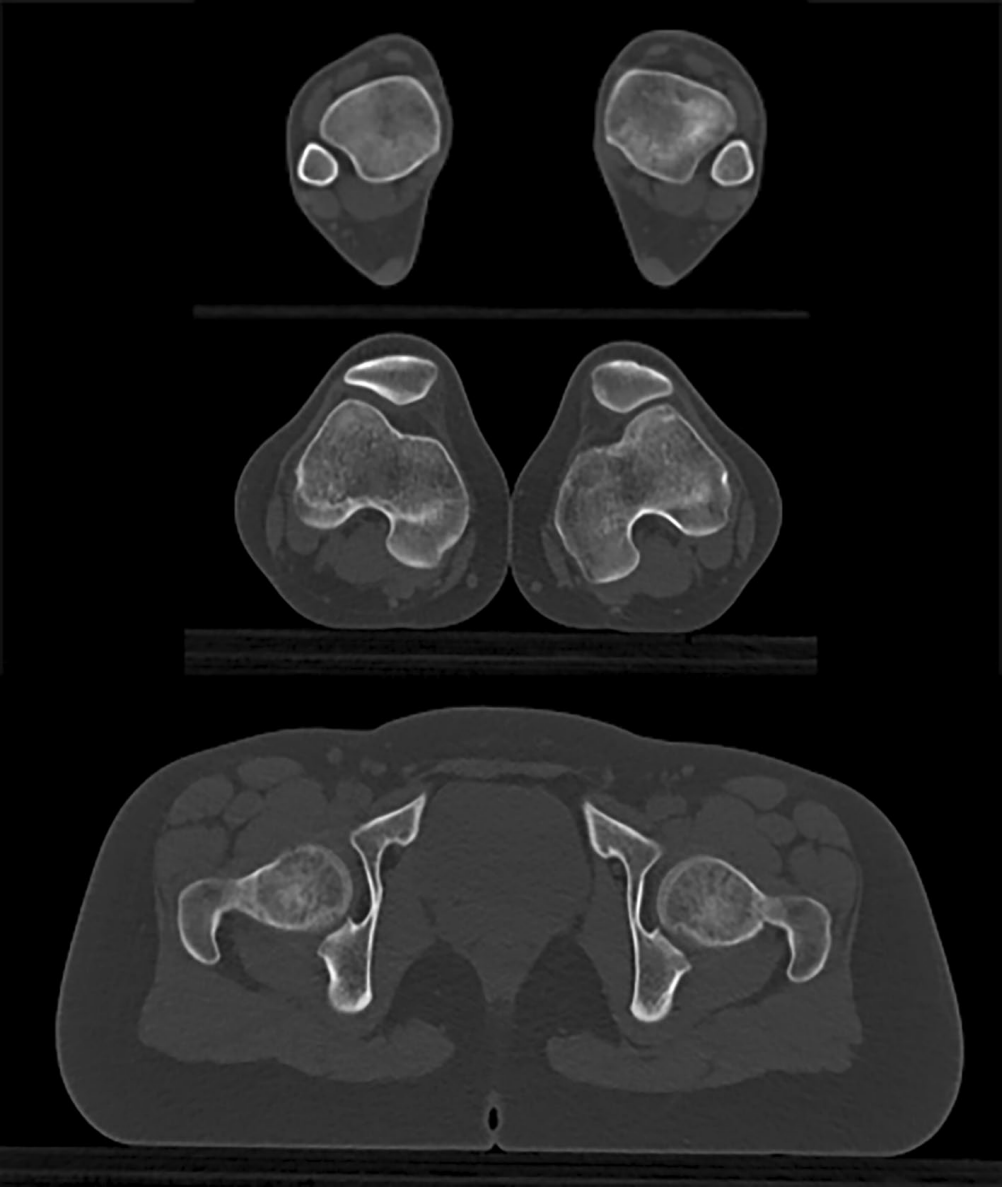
Torsion angle CT scan of a 19 year-old female patient with severe ‘inwardly pointing knee syndrome’. The woman suffered from anterior knee pain and patellar dislocation
